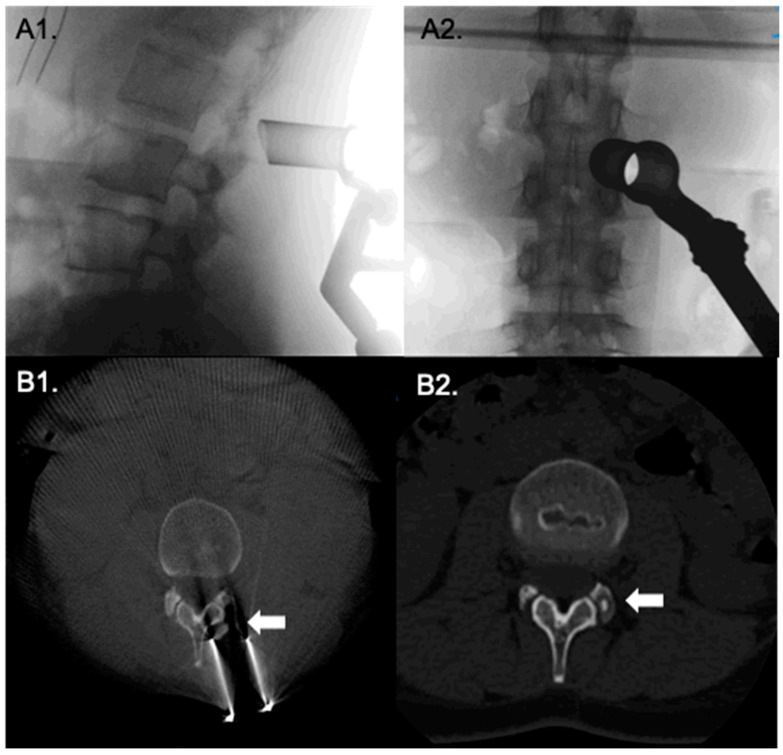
Direct posterior approach to to osteoid osteoma in the facet joint. Intraoperative fluoroscopy, (A1,A2), verify correct positioning of the tubular retractor. (B1,B2) show intraoperative O-Arm CT views before and after tumor removal.
